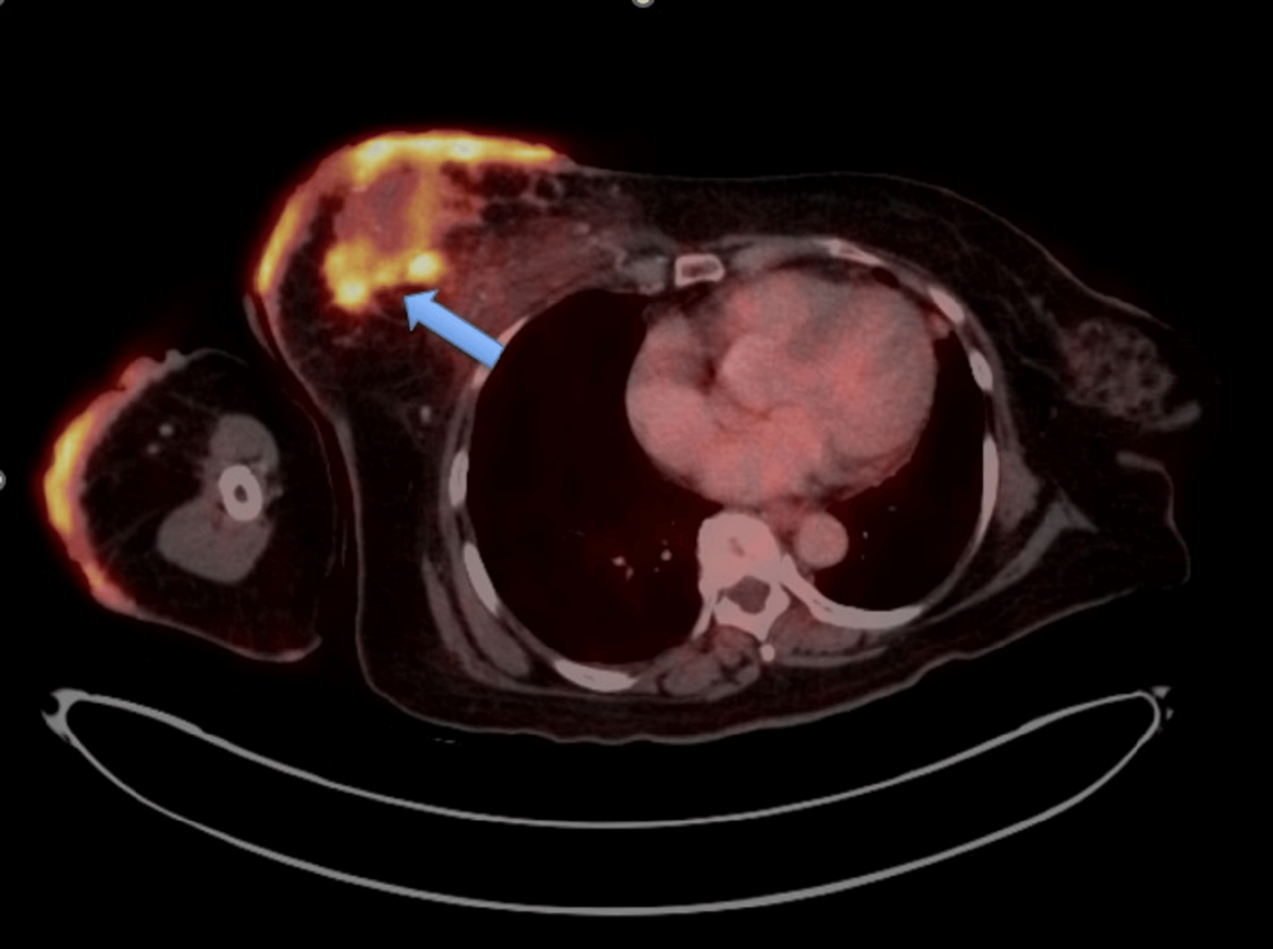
PET scan showing FDG avid contiguous and discrete enhancing soft tissue lesions in the right breast (arrow) PET, Positron emission tomography; FDG, Fluorodeoxyglucose
